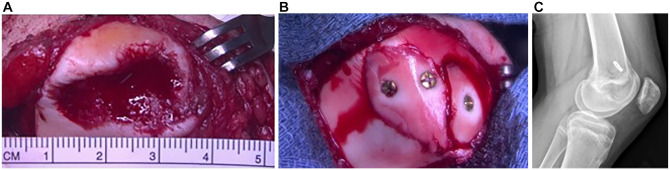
Image of the medial facet of a patellar osteochondral fracture. (A) Site of osteochondral fracture measuring 25 mm in diameter. (B) Osteochondral fragment reduced into lesion site and fixated with 3 headed mini-fragment metallic screws. (C) Lateral radiograph showing screw removal and medial patellofemoral ligament reconstruction that were done 6 months after initial injury, with intact and stable articular cartilage.
